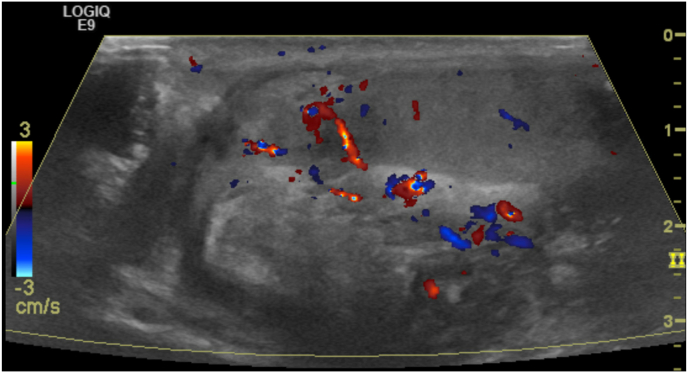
Ultrasound of left testicle
